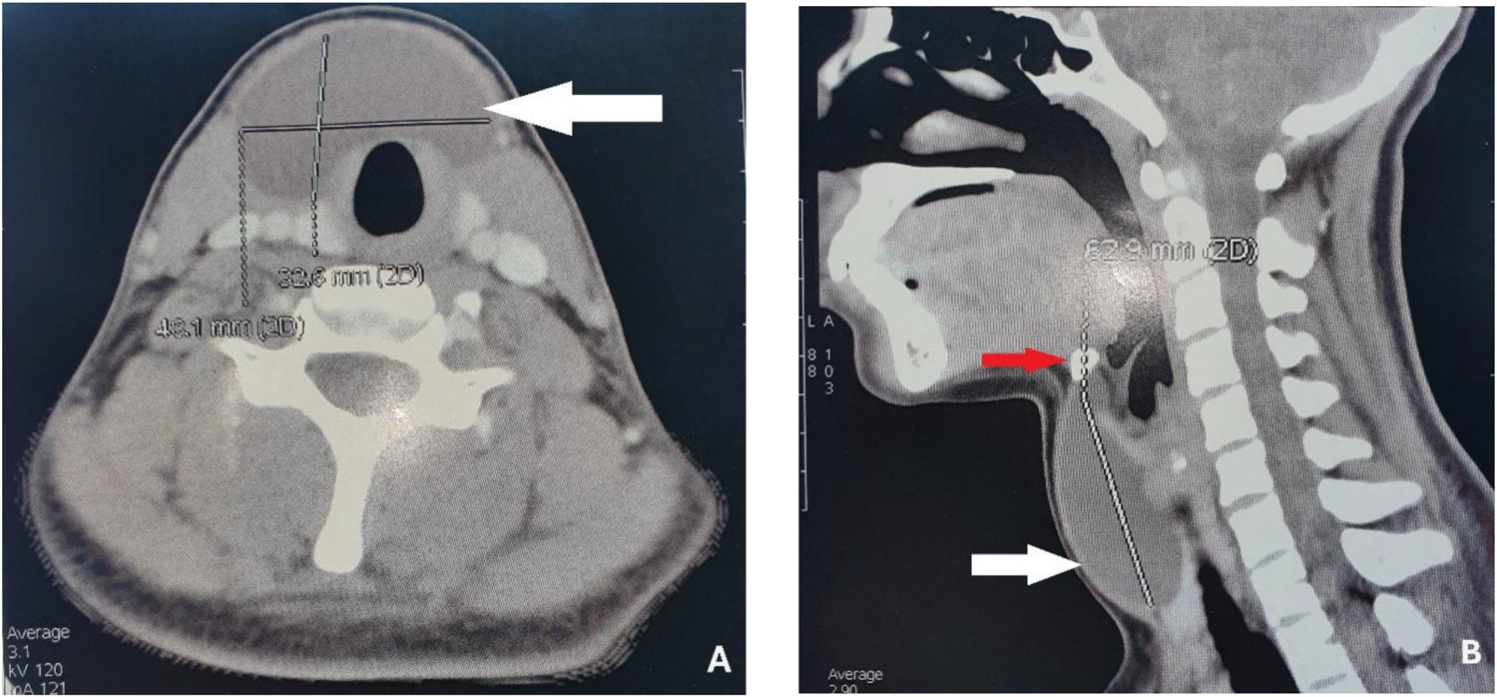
Contrast-enhanced computed tomography of the neck, parenchymal window, axial (A) and sagittal (B) reconstructions, showing a well-defined, homogeneous midline cystic lesion (white arrow) measuring approximately 6.3 cm in maximal diameter, located inferior to the hyoid bone (red arrow). No internal septations, solid components, or post-contrast enhancement are identified.
